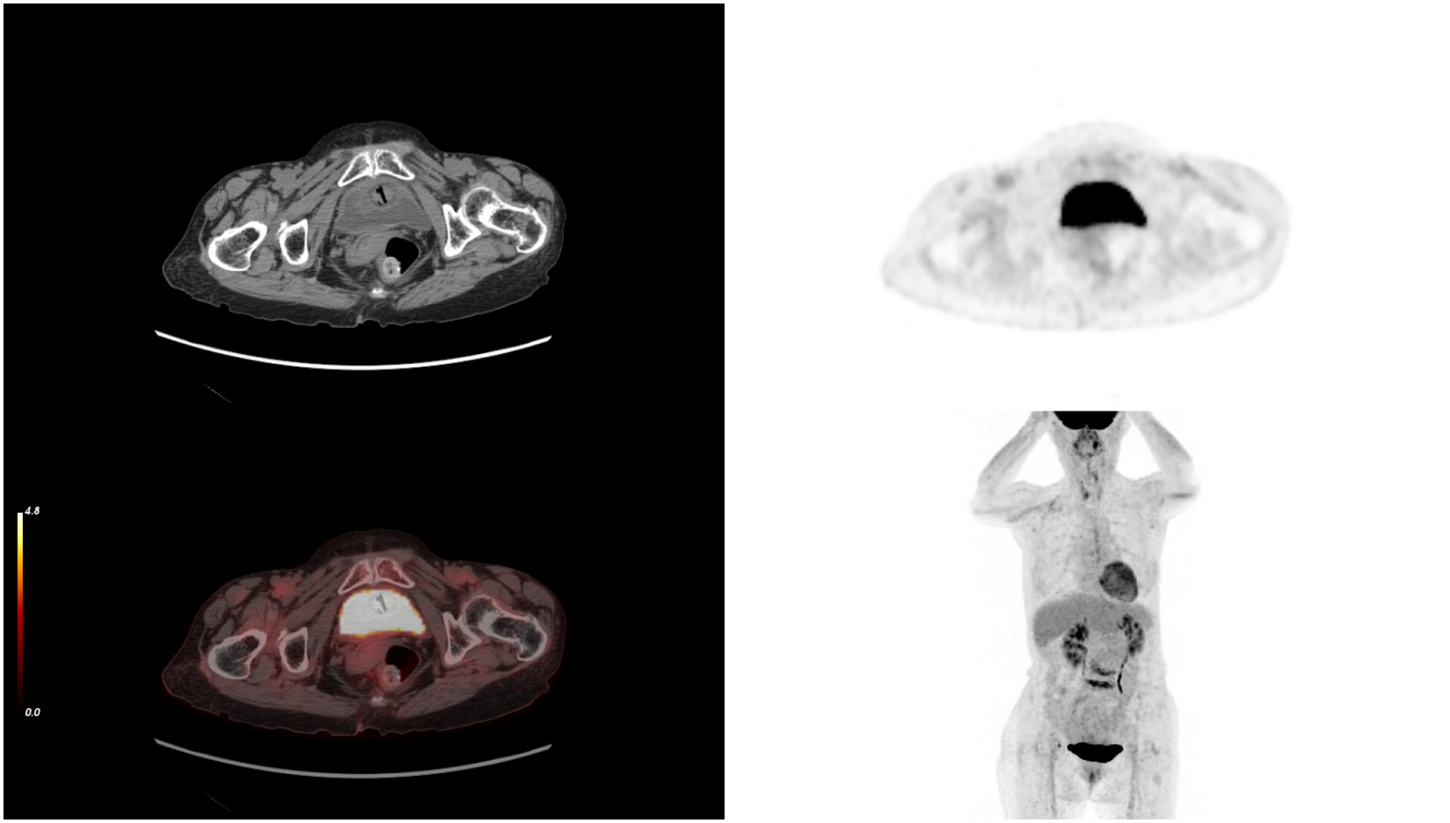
After 10 cycles of treatment, PET-CT results showing the complete disappearance of the tumor. PET-CT, positron emission tomography/computed tomography.
